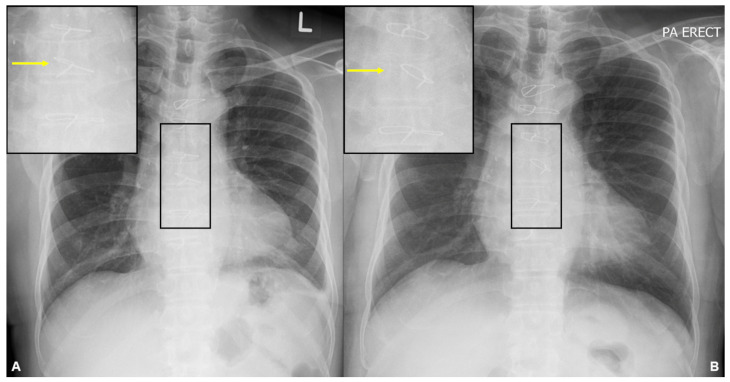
A frontal chest radiograph was obtained on presentation (B), showing left lateral displacement of a sternal wire in a chest radiograph from 3 years ago (A). No fracture of the sternal wires is seen. Initial laboratory investigations showed a low haemoglobin level of 8.8 g/dL. The platelet and white cell counts were within normal limits. The serum creatinine level was elevated (149 µmol/L; estimated glomerular filtration rate of 42 mL/min) with normal serum calcium and electrolyte levels. The liver function tests were normal, and the serum alkaline phosphatase (ALP) and lactate dehydrogenase (LDH) levels were not elevated.
